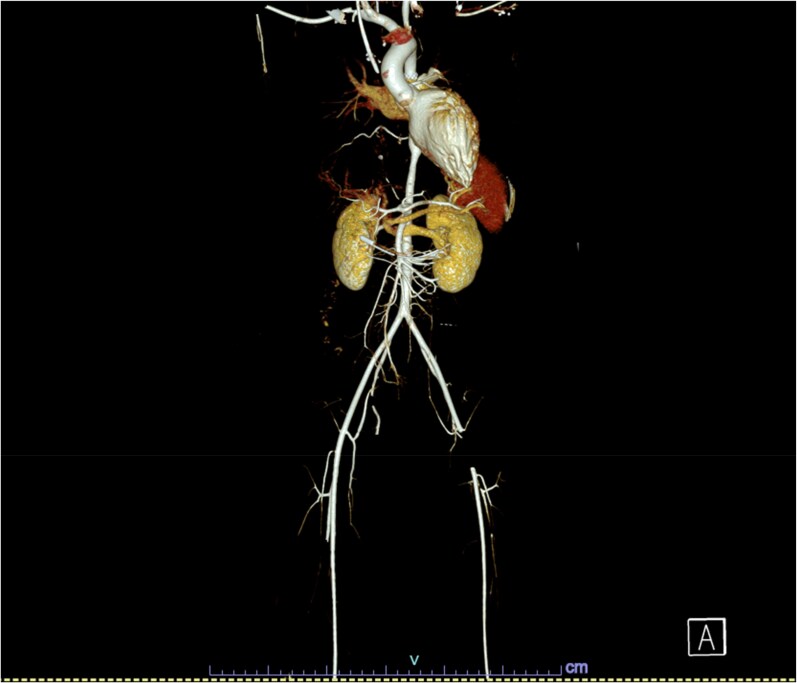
The 3D reconstruction of computed tomography angiogram images (pre-aspiration mechanical thrombectomy) showing thrombosis of left common femoral artery, with visible aortic stents after coarctation of aorta stenting.
